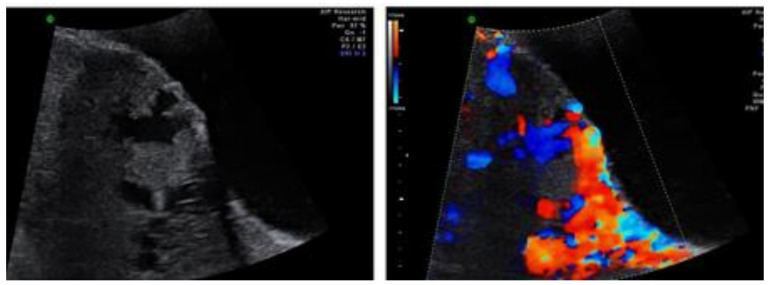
PAS lacune in color Doppler ultrasound (right) and grey-scale ultrasound (left). Imaged adopted from Adu-Bredu et al. [36].
